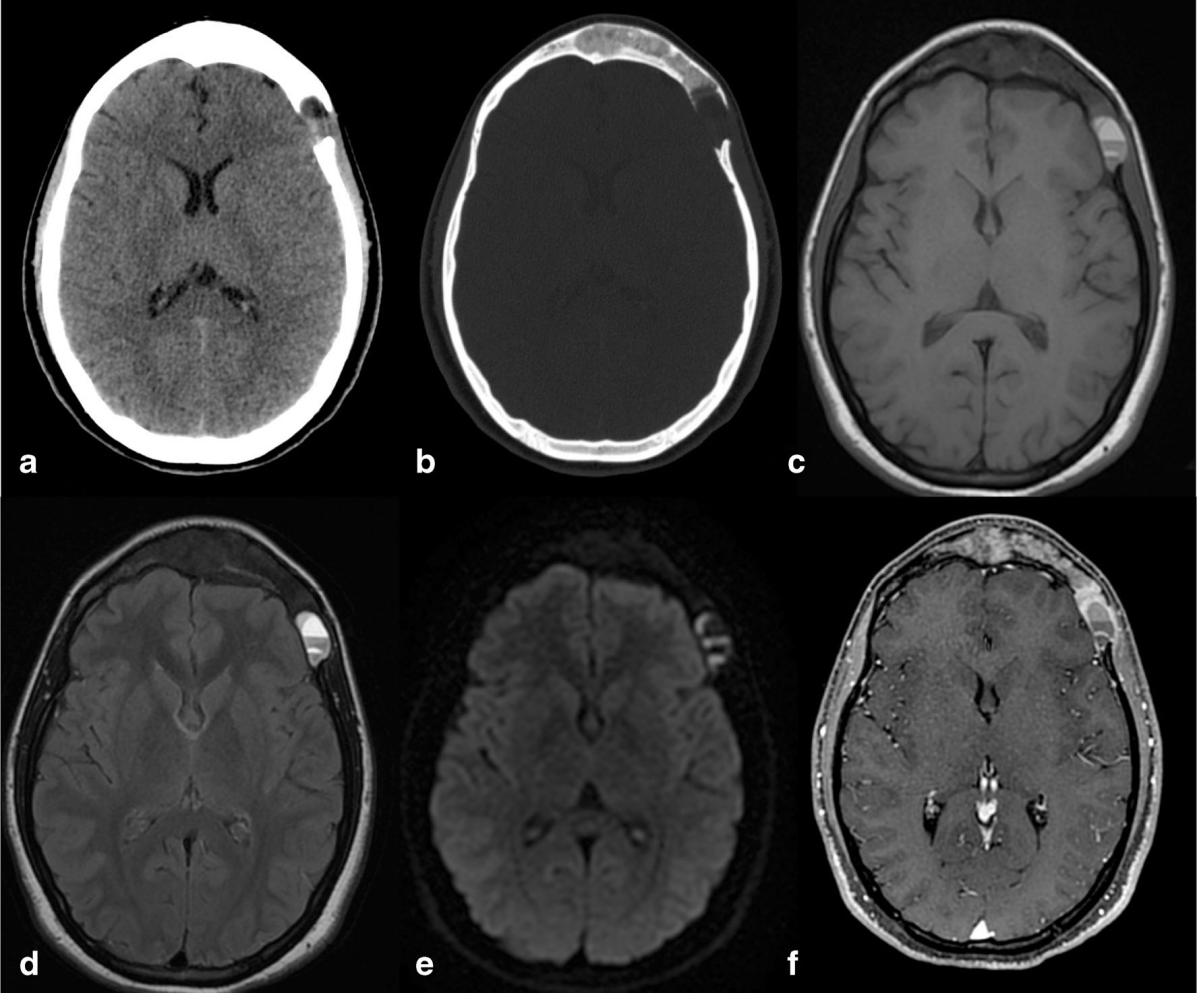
Aneurysmal bone cyst (ABC). Axial CT images with brain (a) and bone (b) windows show a left frontal lytic lesion with blood-fluid levels, associated with a diffuse ground-glass modification of the frontal bone, related to the presence of a fibrous dysplasia. Magnetic resonance (MR) examination better depicts the multiloculated lesion, confirming blood-fluid levels on T1w (c), FLAIR (d) and DWI (e) images, and showing enhancement of the capsule and internal septa (f)
